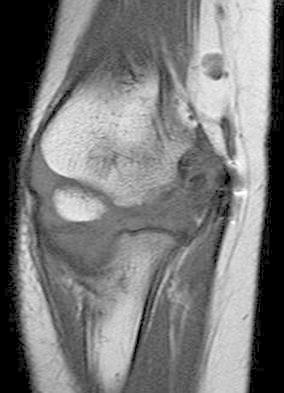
Six months after surgery, a T1-weighted spin-echo magnetic resonance imaging (repetition time/echo time 820 ms/96 ms) showed a well-positioned and united cartilaginous trochlea
